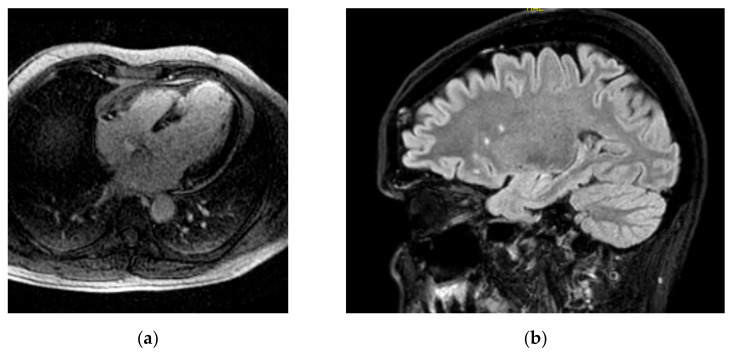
Combined brain/heart MRI images from a patient with CD and HF (a) inversion recovery image showing extensive myocardial LGE due to myocardial infarction; (b) brain FLAIR image showing WMH in the same patient.
